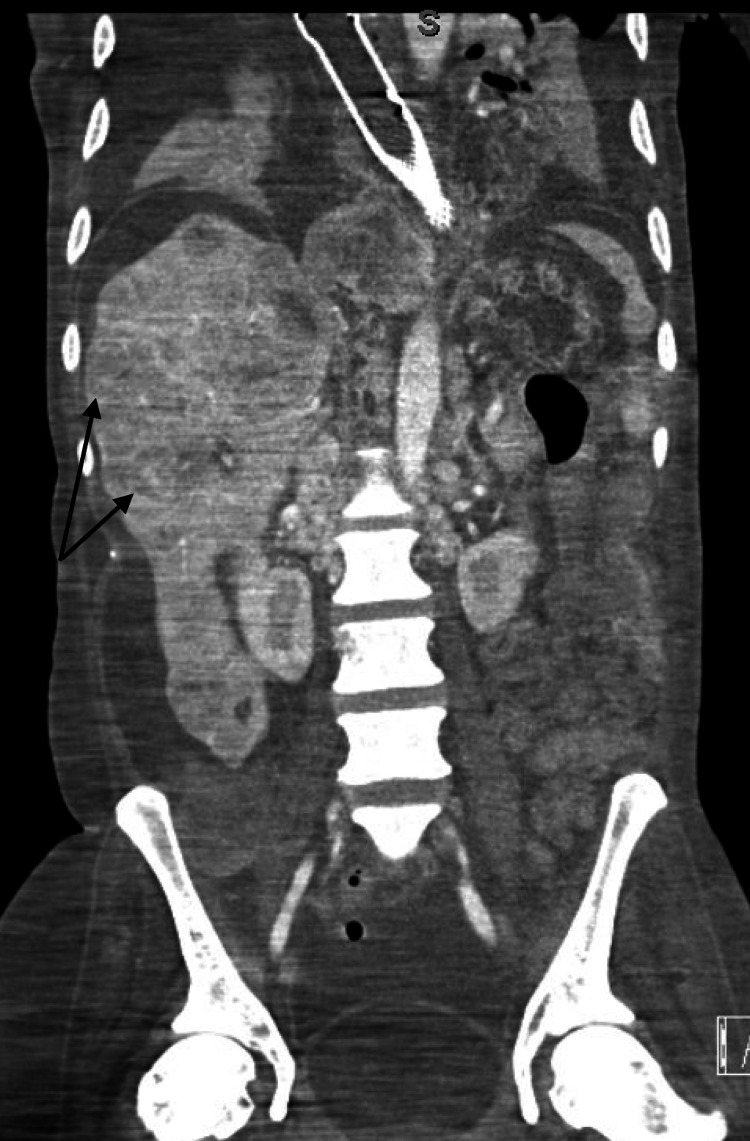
Contrast CT chest/abdomen with arrows showing multiple hepatic metastases
